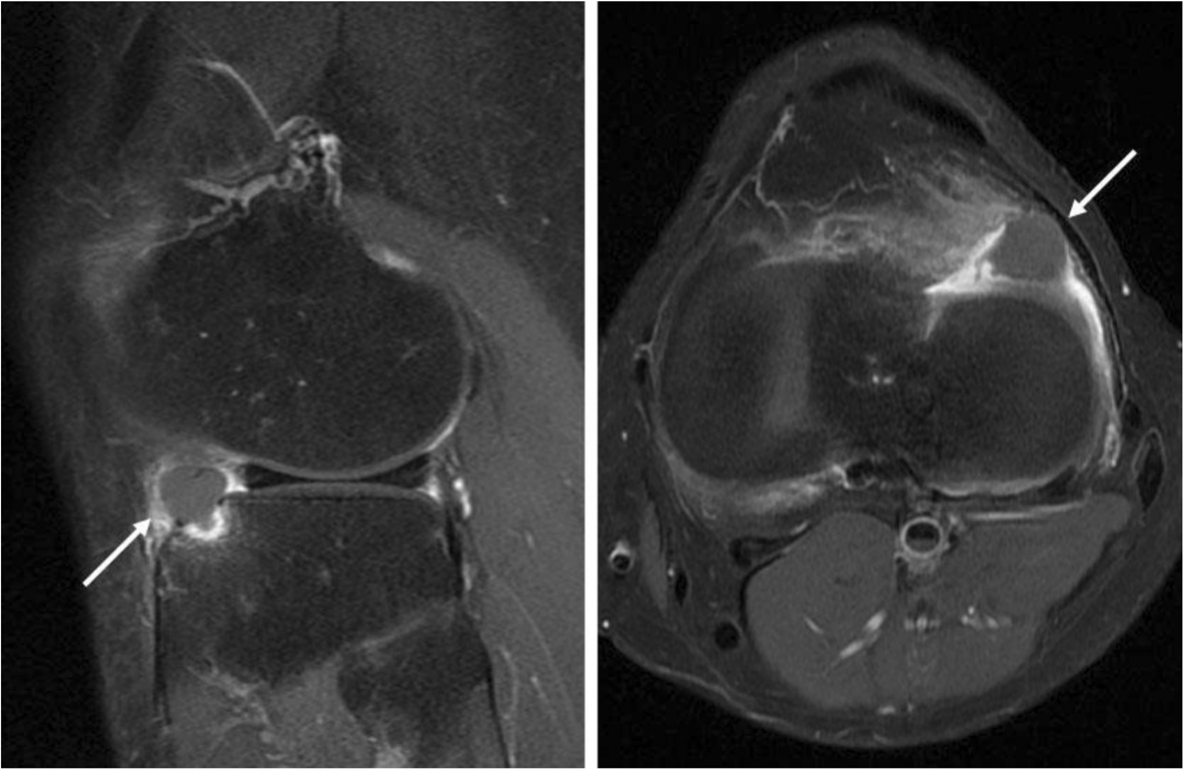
Gadolinium enhancement with fat saturation magnetic resonance images. A homogeneously isointense lesion is identified on both sagittal and axial sections of the images (arrows). The mass is enhanced only in the peripheral area. Gadolinium-enhanced magnetic resonance imaging, repetition time/echo time = 558/10, field of view = 160×160mm, slice thickness = 4mm.
